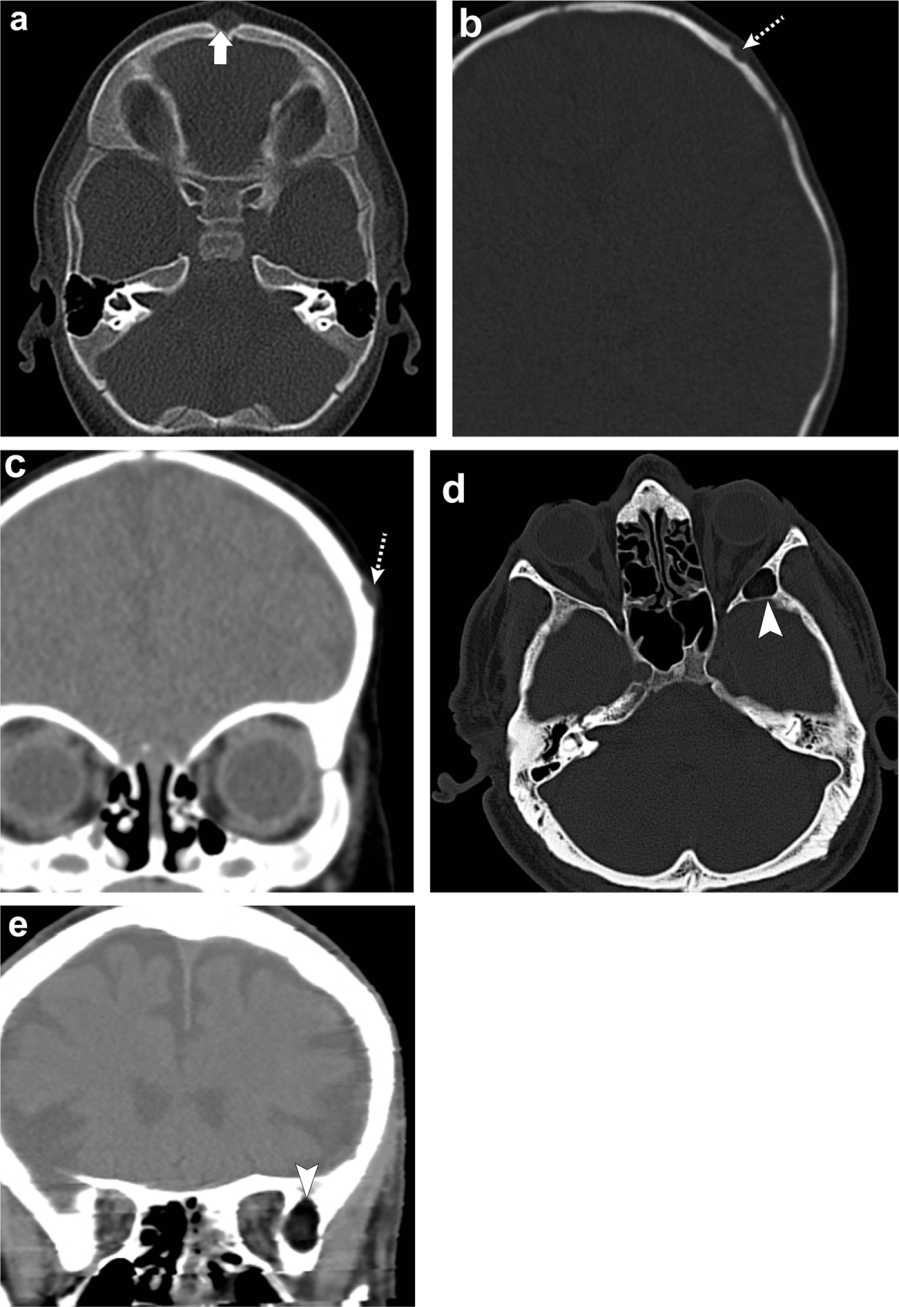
Dermoid cysts. *Patient 1:* Axial head CT (a) depicts a midline fat-containing lesion in the frontal region (*thick arrow*). *Patient 2:* Axial bone window (b) and coronal soft tissue window head CT (c) show a dermoid cyst in the left frontal bone involving the outer table (*dashed arrows*). *Patient 3:* Axial CT (d) and coronal head CT portray a fat-containing lesion in the left grater wing of the sphenoid (*arrowheads*)
